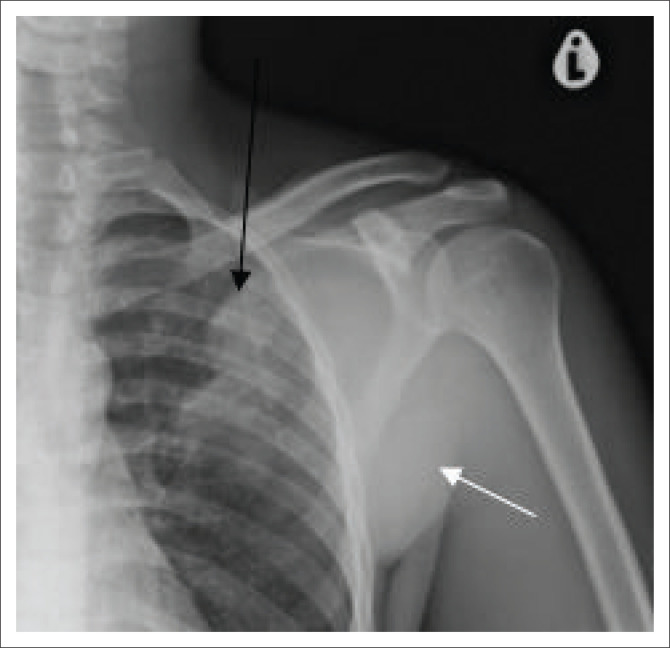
Frontal radiograph of the left shoulder: Left axillary soft tissue prominence (white arrow). Left upper lobe air space disease, with early internal cavitation (black arrow).
